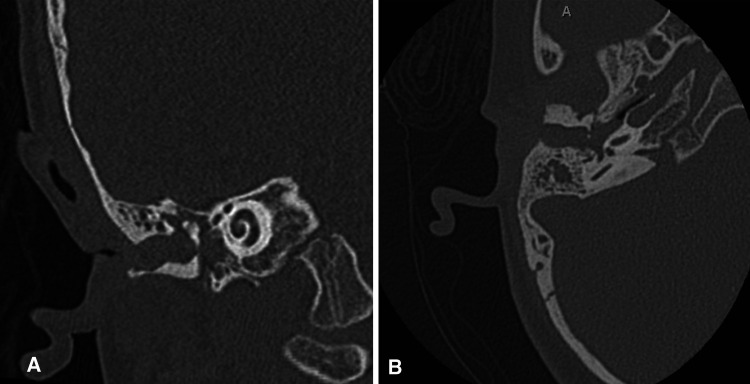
(A) Coronal and (B) axial non-contrast temporal bone CT scan demonstrating severe EAC stenosis with cholesteatoma.
